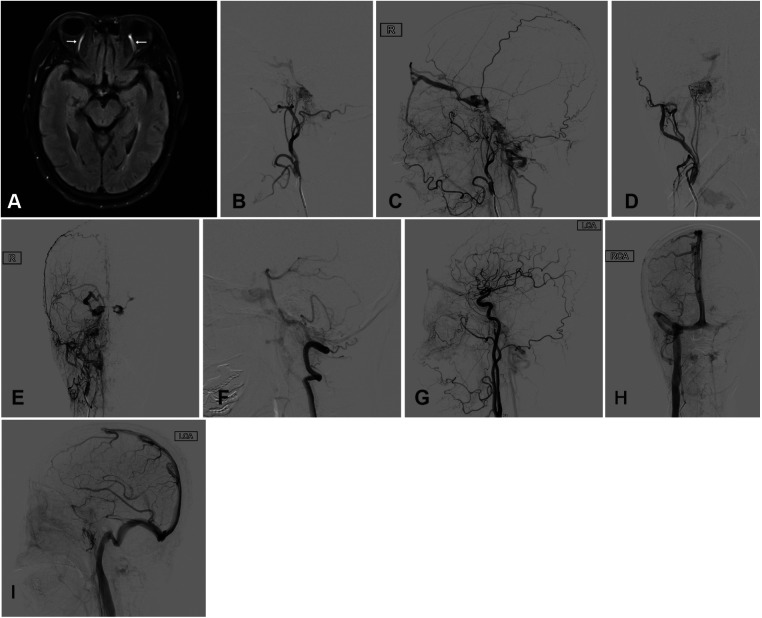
(A) MRI revealed mild dilation of bilateral superior ophthalmic veins (white arrows). Angiography of the right external carotid artery (B–E), right vertebral artery (F) and the left common carotid artery (G) showed the right HCDAVF, which was drained into the cavernous sinus through IPS vein, then entered the facial vein through the right ophthalmic vein, and finally reached the external jugular vein. HCDAVF, Dural arteriovenous fistulas of the hypoglossal canal. The patient's right internal jugular vein is not occluded (H) and the left IPS was occluded (I). IPS, inferior petrosal sinus.
